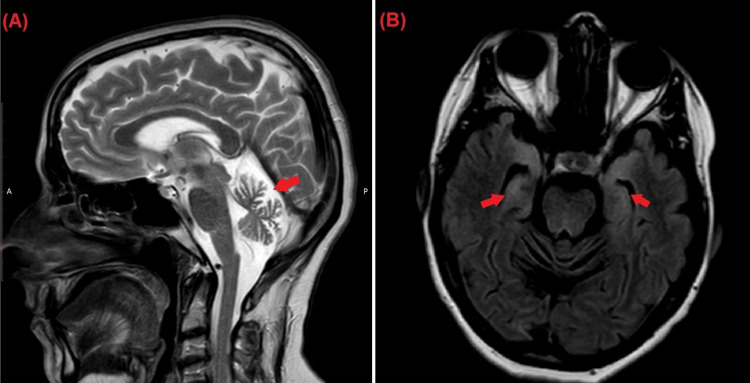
Sagittal T2-weighted images MRI (A) shows significant cerebellar atrophy manifested by volume loss and widening of the CSF space (arrow); Axial FLAIR MRI (B) shows bilateral asymmetrical increased signal intensity of both mesial temporal lobes (arrows). MRI, magnetic resonance imaging; CSF, cerebrospinal fluid
